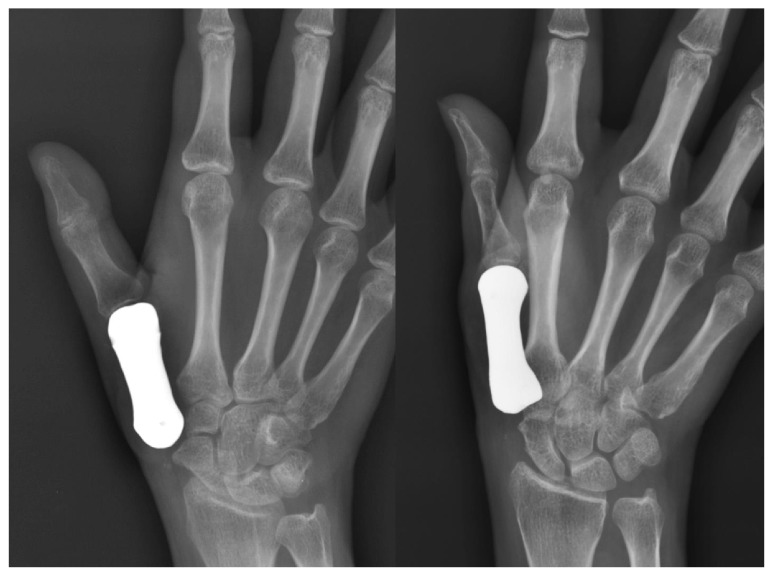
Plain radiographs of a patient-matched total first metacarpal prosthesis.
